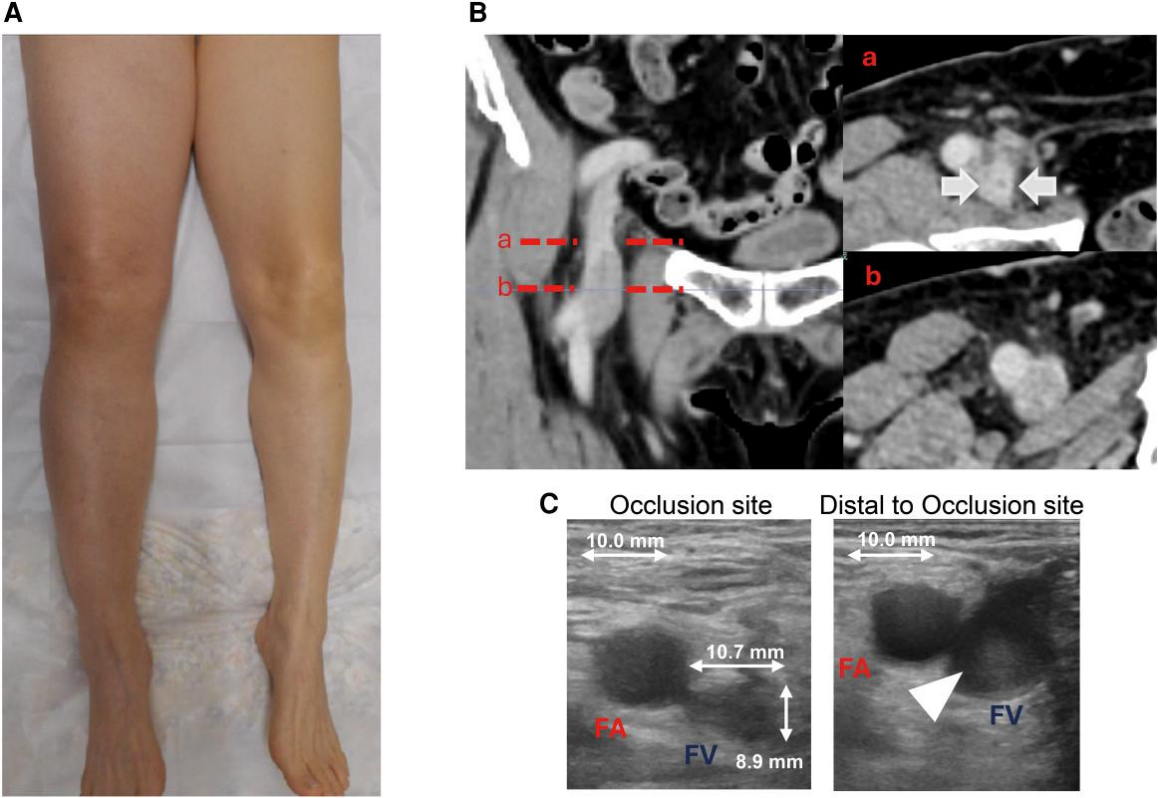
Clinical and imaging findings on Day 12. (*A*) Physical examination showing diffuse swelling and mild erythema in the right lower extremity. (*B*) Contrast-enhanced computed tomography (CT) demonstrating narrowing of the right common femoral vein (CFV) with peripheral venous dilation. Axial slices at two levels (a, b) are shown on the right. White arrows indicate the narrowed CFV lumen. (*C*) Duplex ultrasonography showing narrowing of the right CFV. The left panel (occlusion site) demonstrates the vessel dimensions (10.9 mm × 9.4 mm), while the right panel (distal to the occlusion site) reveals a hyperechoic lesion (arrowhead) within the vein, suggestive of thrombus. FA, femoral artery; FV, femoral vein.
